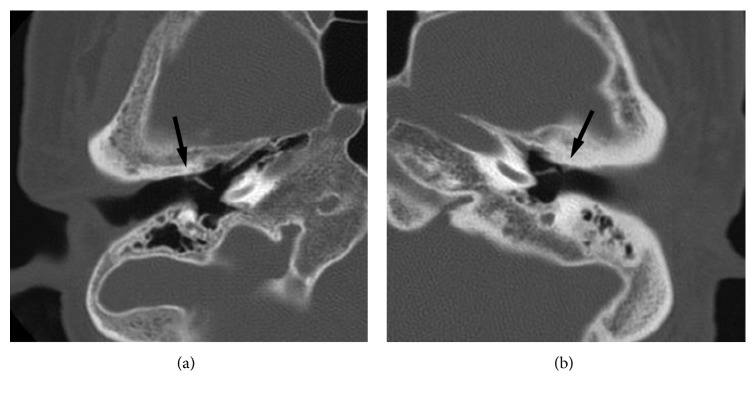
CT images of the temporal bone in Case 1 (a) and Case 2 (b). CT imaging shows a lesion emanating from the anterior bony EAC in both cases. In each case, the EAC appears flat with well-defined boundaries. Arrows indicate the masses.
